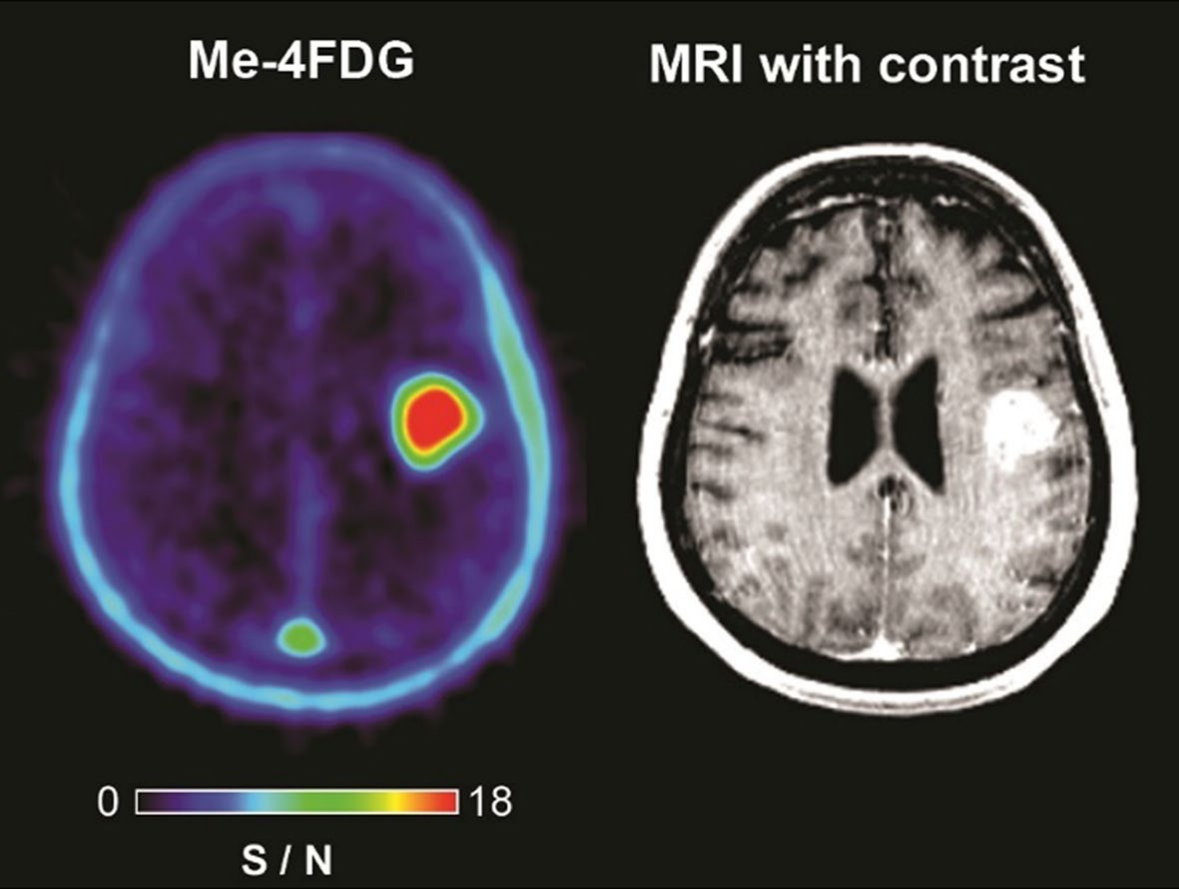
Me-4FDG PET and MRI scans on a WHO Grade IV astrocytoma patient. The Me-4FDG S/N (SUVR/BG) scale is for the NIH color scale
